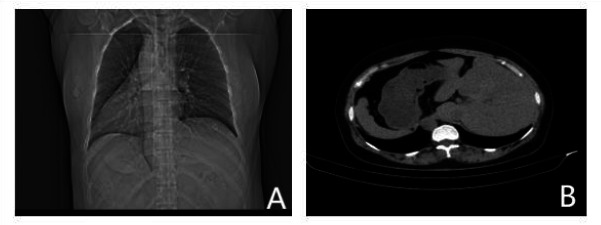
Shows that CT confirmed the patient’s SIT: (A) shows the right side of the heart on a chest CT scan; (B) shows the left and right internal organs inverted.
